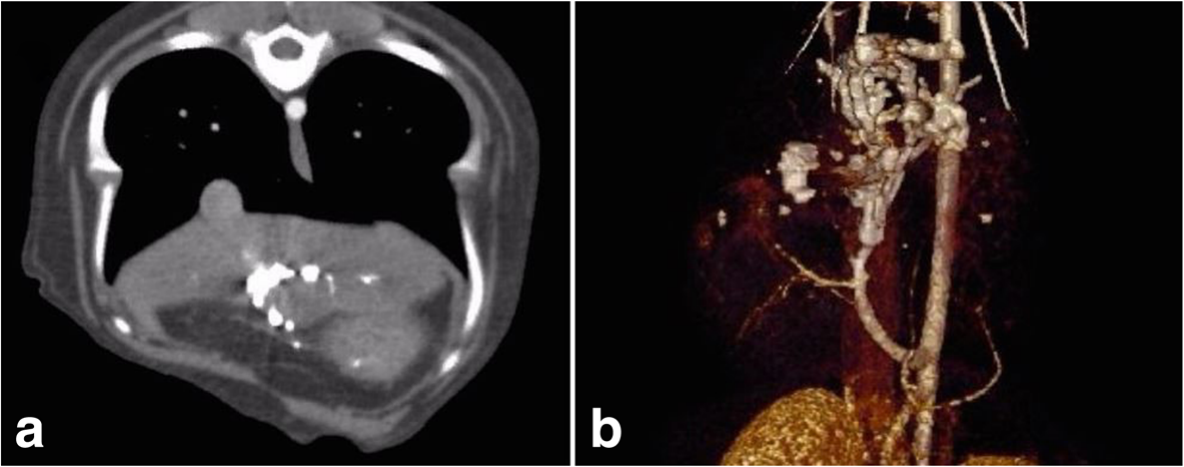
Post procedural CTA in Patient 1a) Dense PHIL cast within the gallbladder fossa without residual arterial flow. b) 3D volumetric reconstruction shows the PHIL embolic cast without evidence of recurrent or residual AVM
